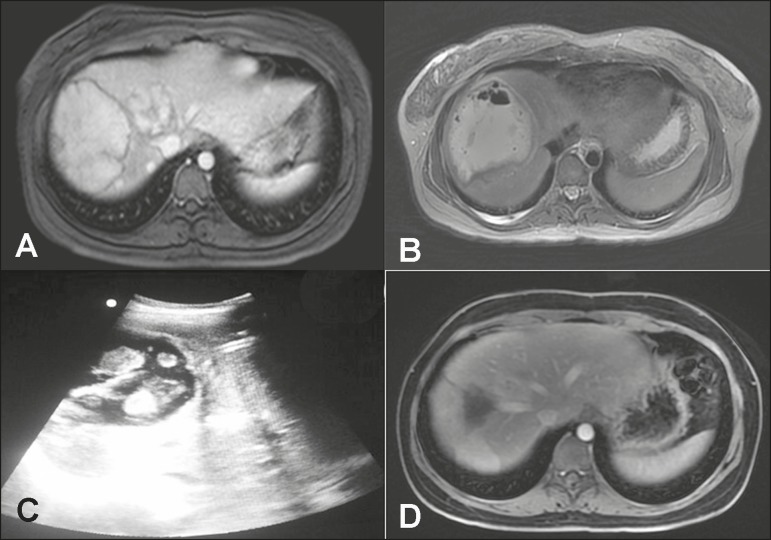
A: Abdominal MRI performed prior to embolization, showing a
